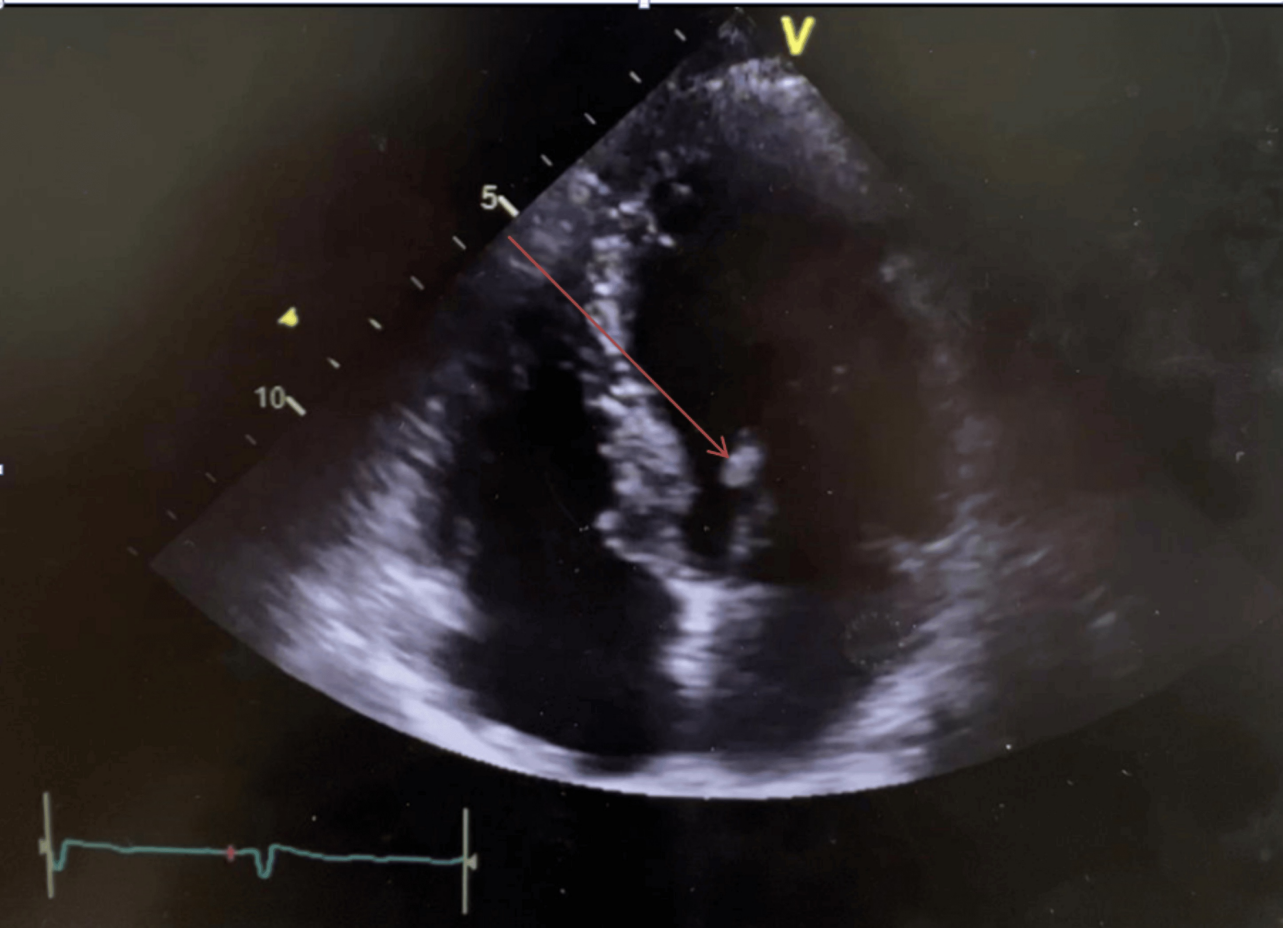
Apical four-chamber view showing an echogenic mass attached to the anterior mitral leaflet, oscillating within the left ventricle (arrow).
